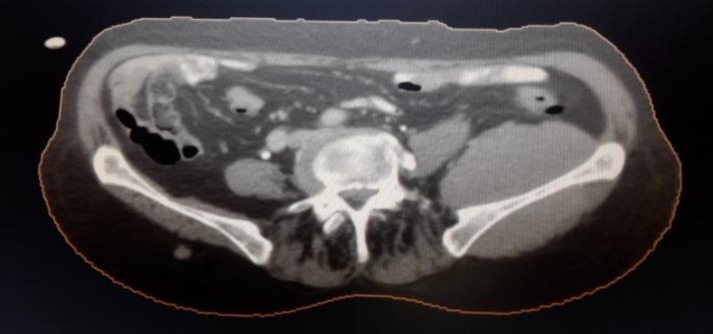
CT scan showing huge left paraspinal mass on the left side
